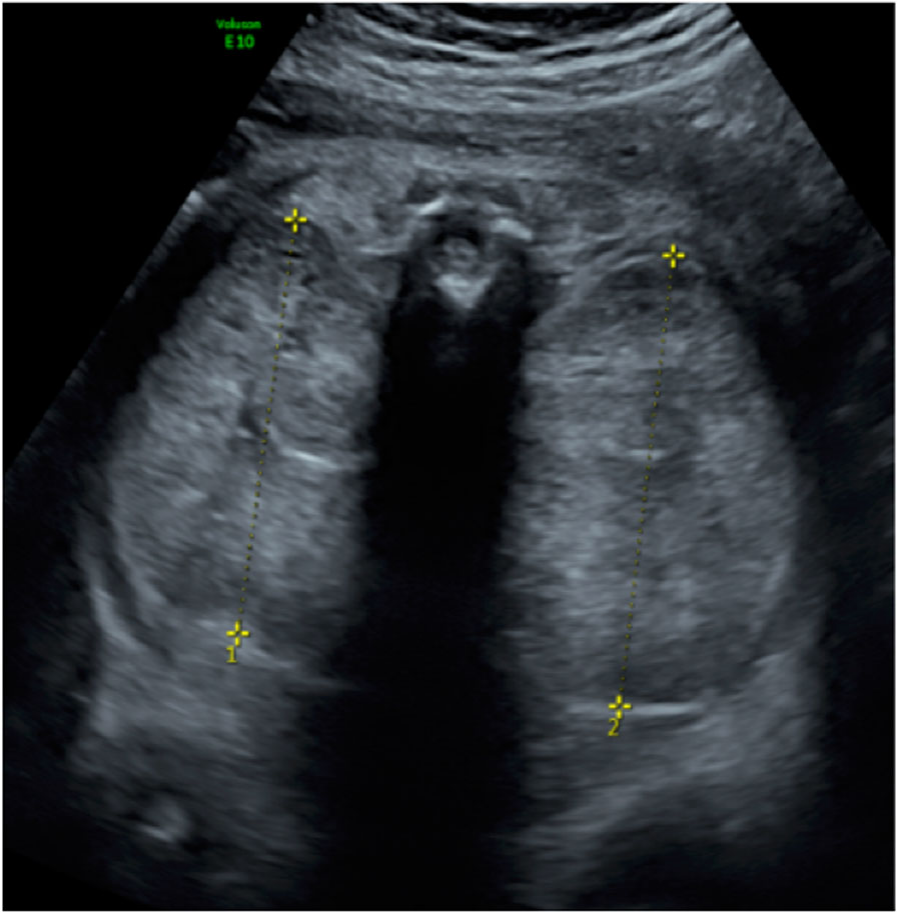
Ultrasound at 35 weeks of gestation. Right kidney measures 65 × 90 × 63 mm; left kidney measures 70 × 78 × 75 mm
